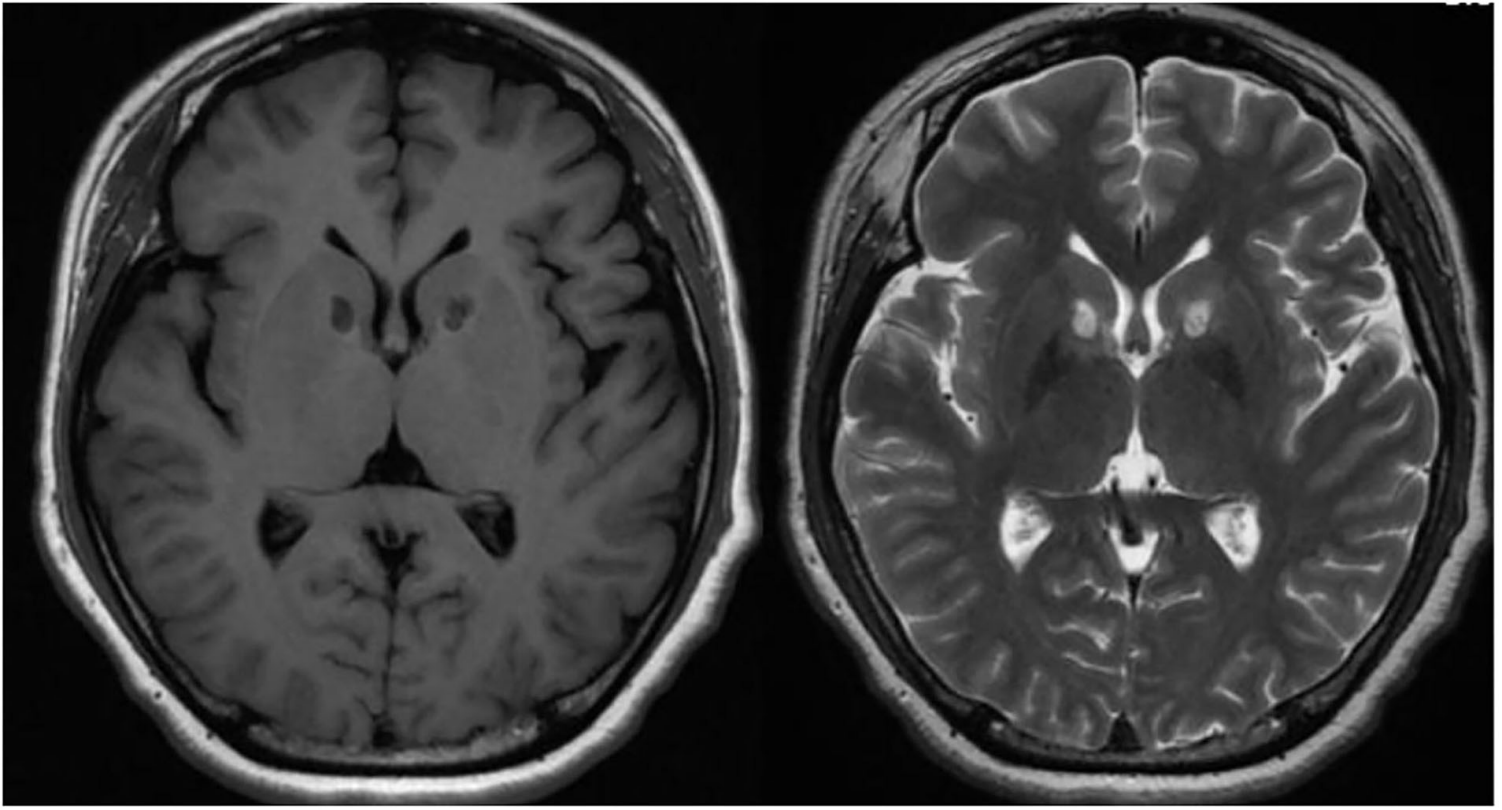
One-week post-operative MR image of a patient with MRgFUS Capsulotomy.
